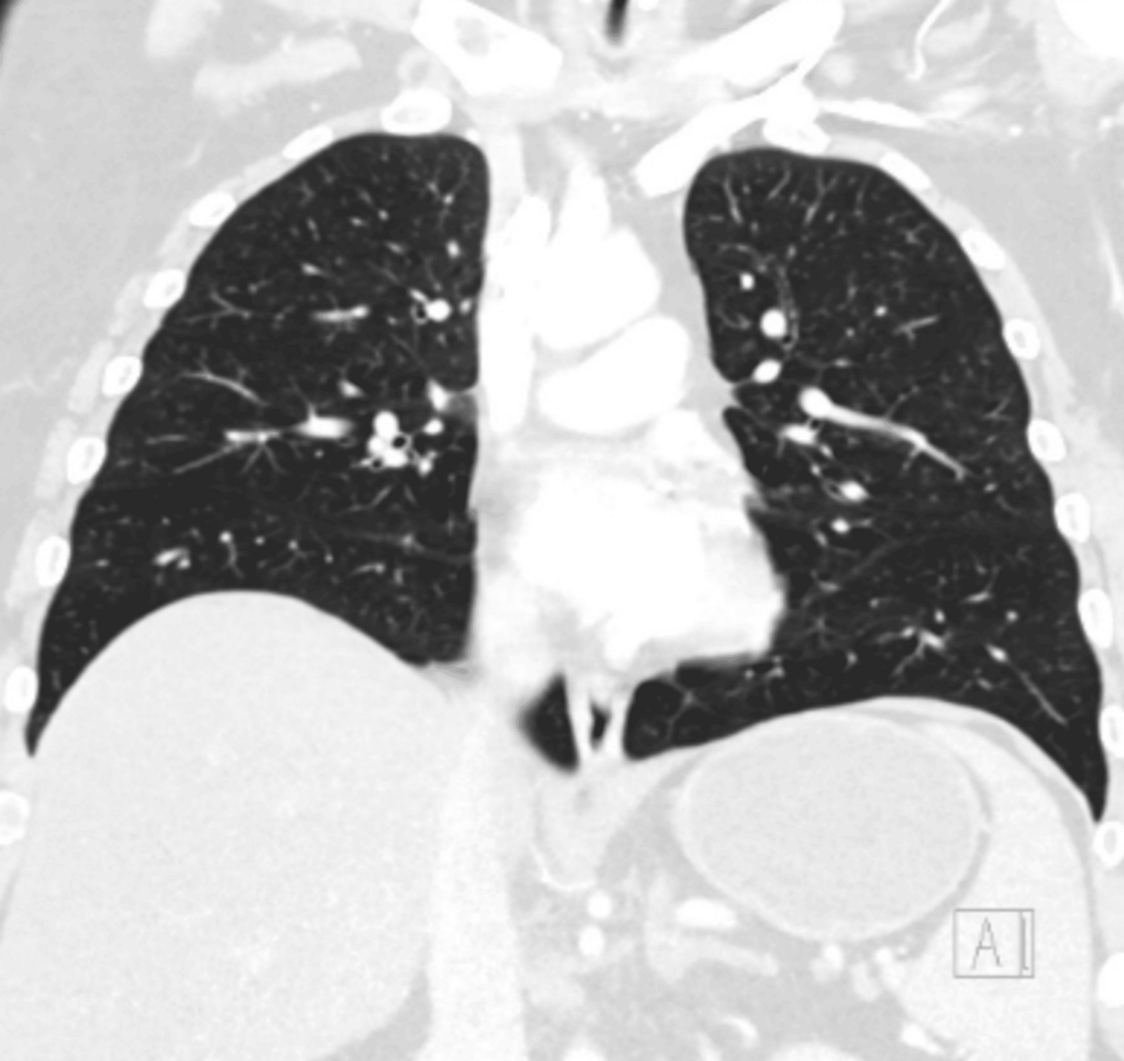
CT chest at admission with no pulmonary consolidation, nodule, mass, effusion, and no acute abnormality noted
